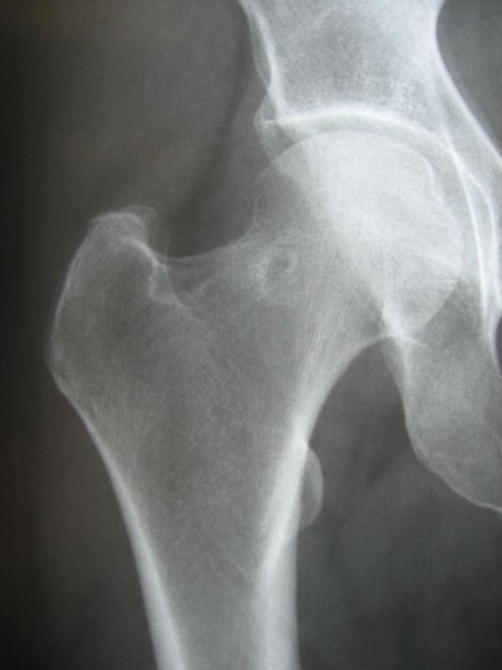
Plain radiograph showing an oval nidus surrounded by a radiolucent ring at the femoral neck proximal to the femoral head of the right hip 6 months after RF ablation.
